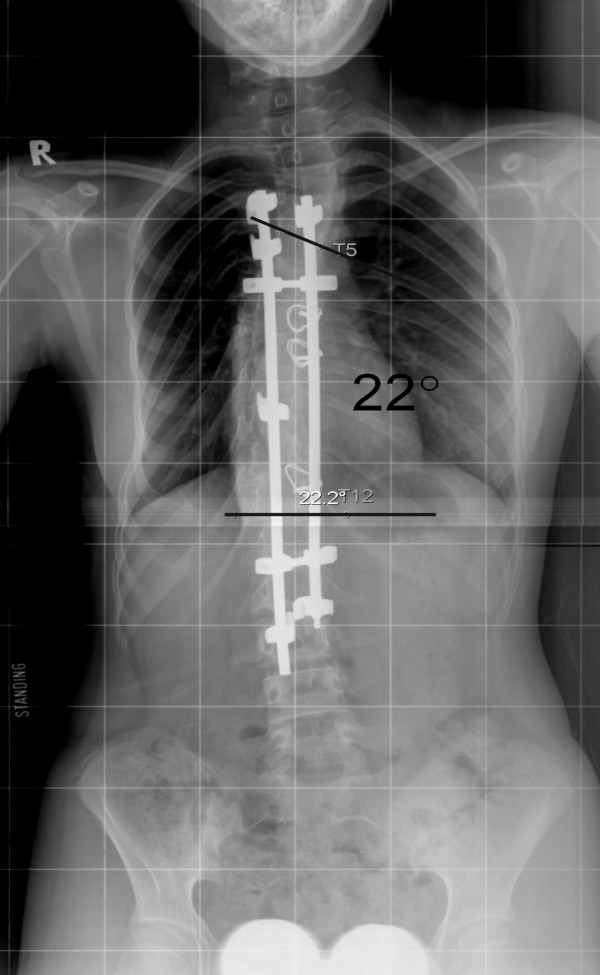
Standing radiograph after posterior instrumentation.
